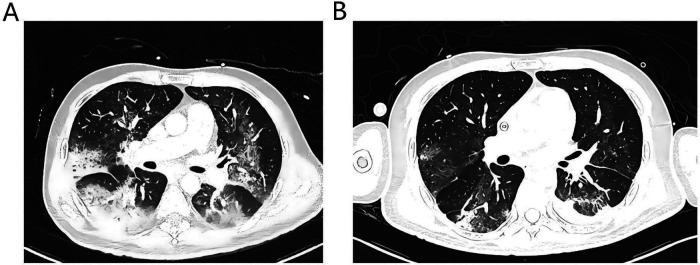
Comparative chest CT scans: (A) preoperative CT scan showing extensive bilateral pulmonary edema and consolidation. (B) CT scan on the eighth day of extracorporeal VAD support showing significant resolution of pulmonary edema and consolidation.
